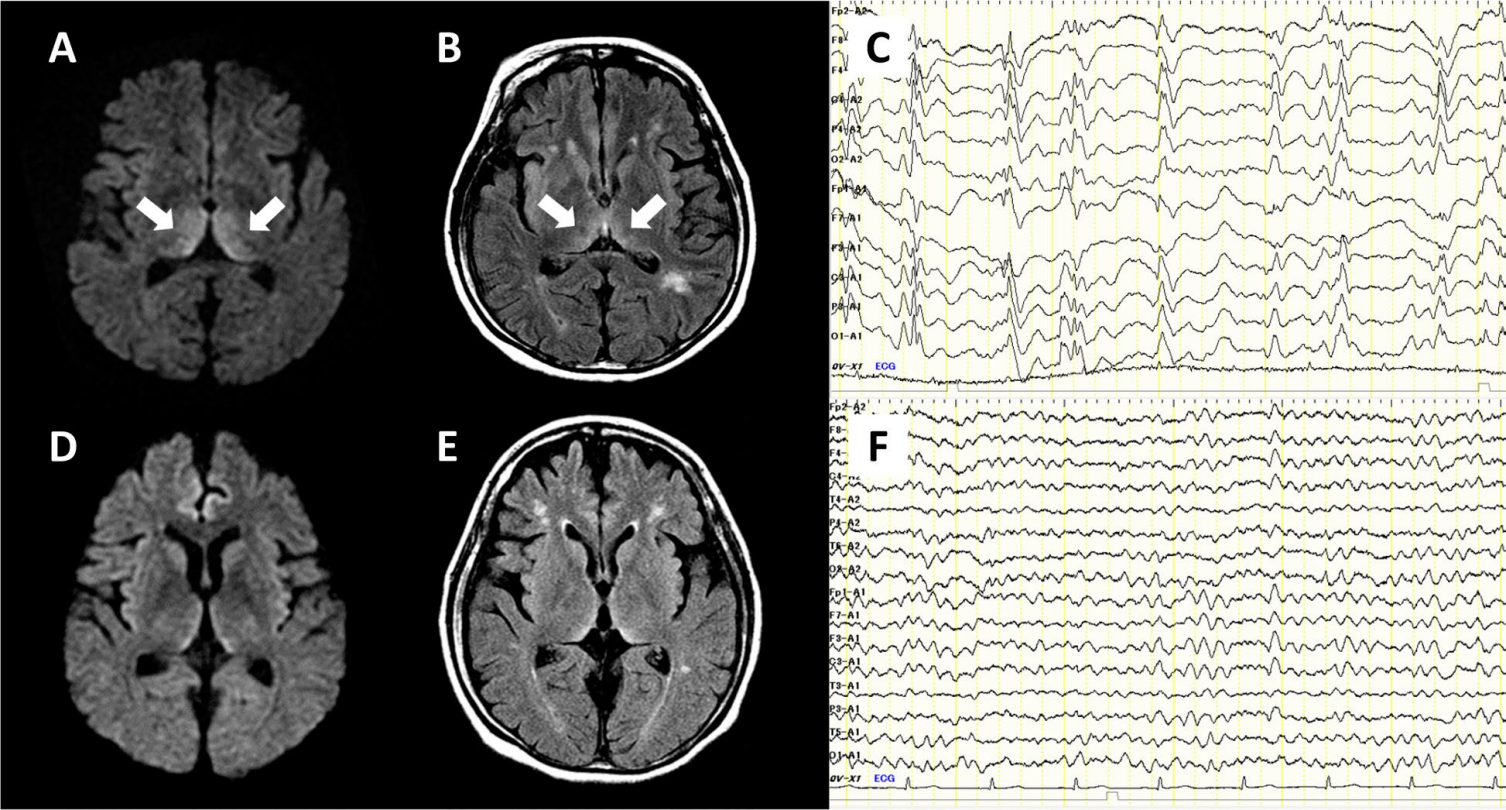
Brain magnetic resonance imaging and electroencephalography findings of Patient 2. Magnetic resonance image (A: diffusion-weighted imaging, B: fluid-attenuated inversion recovery imaging) obtained in the acute phase shows high-intensity lesions in the medial thalamus (arrows). Electroencephalogram obtained in the acute phase shows generalized periodic discharges with polyspikes (C). The findings of Patient 2 are similar to those of Patient 1, and they improved in the chronic phase (D-F)
